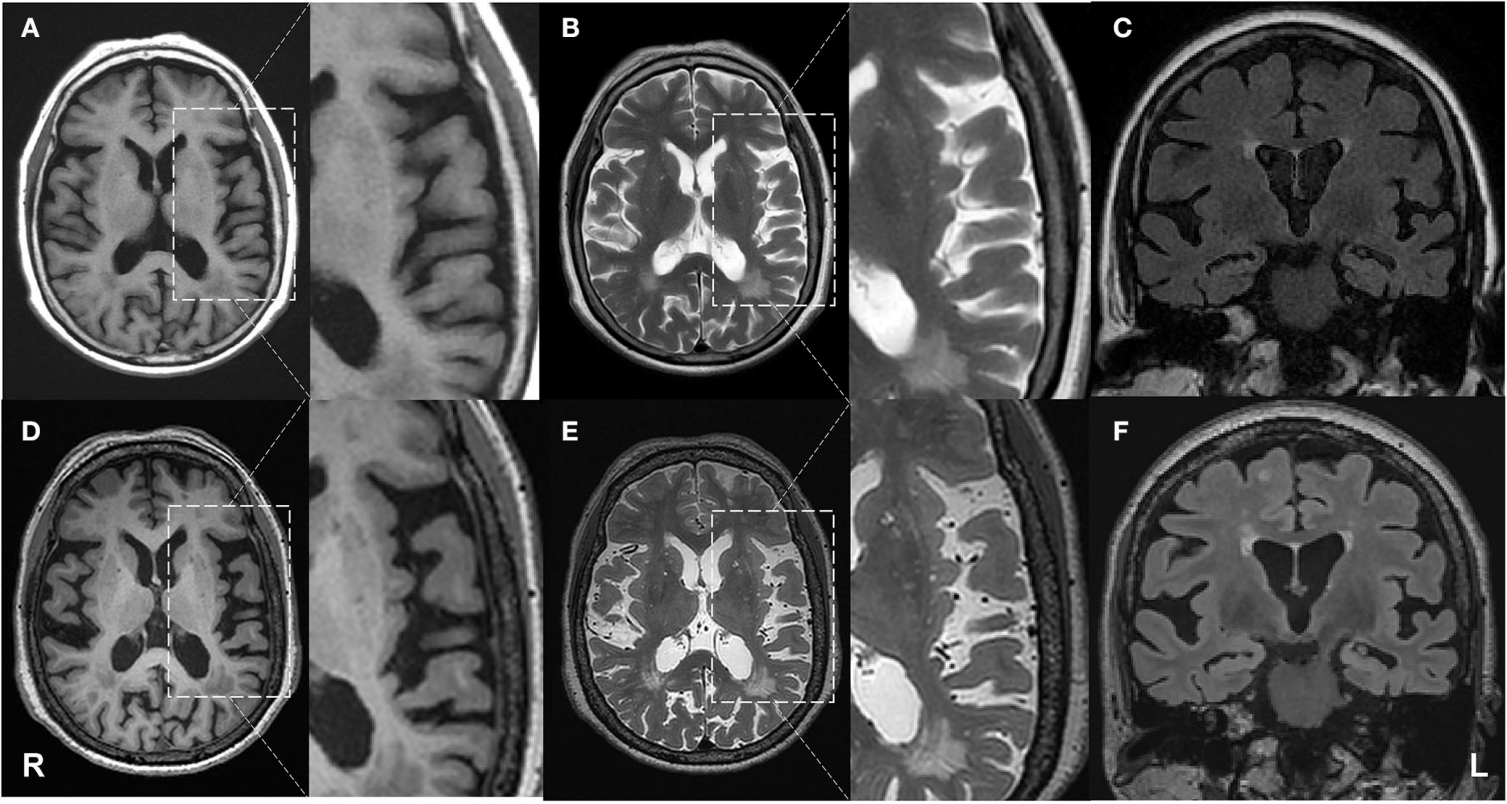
Progressive brain atrophy was detected by structural MRI. First row: MRI on 15 February 2019; second row: MRI on 25 April 2021. (A,D) axial T1 weighted images; (B,E) axial T2 weighted images; (C,F) coronal FLAIR images. The patient showed atrophy in the left temporal lobe compared with the baseline.
